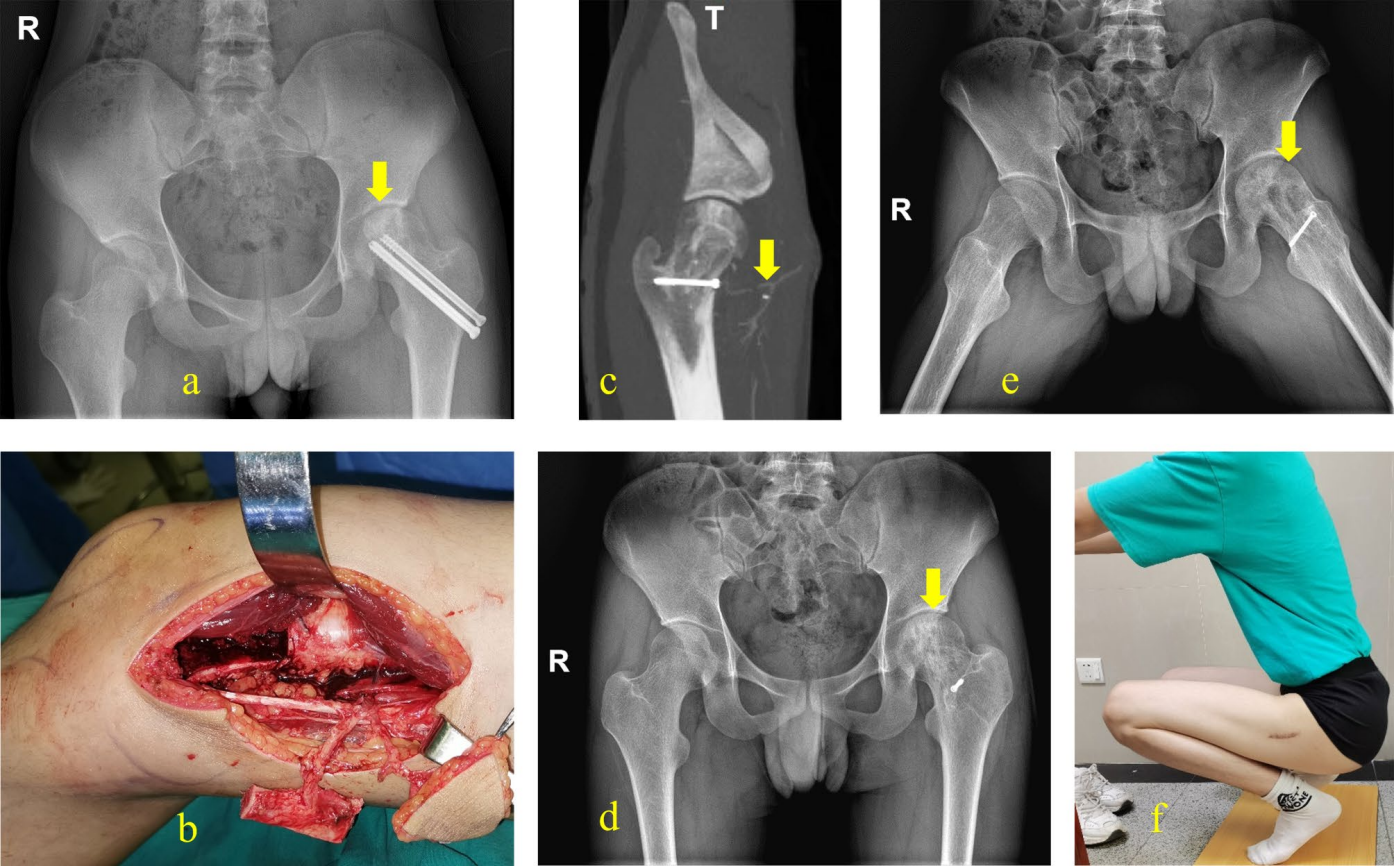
Case 3. Anteroposterior radiograph of the pelvis shows cystic degeneration (yellow arrow) in the femoral head(a).Intraoperative picture shows the harvested chimeric DGA flap with the pedicle(b). Postoperative CTA showed that the vascular anastomosis of the flap(yellow arrow) was unobstructed(c). After a follow-up of 48 months, there is no abnormality in the shape of the femoral head and the cystic degeneration has disappeared. (d, e). Final appearance of hip range of motion (f)
